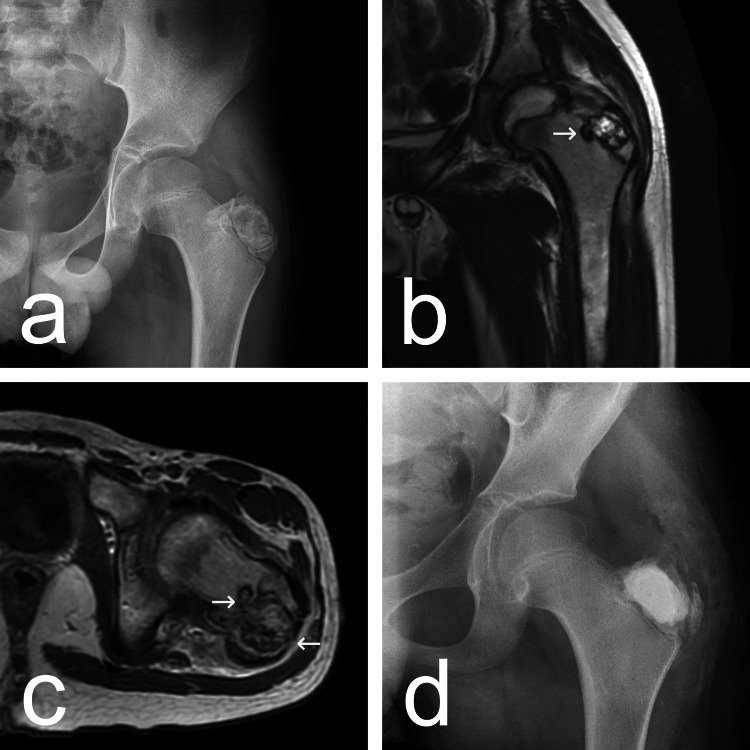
Imaging studies before and after surgery. 1a: Plain radiograph (anteroposterior) of the proximal femur at presentation. 1b, 1c: Frontal oblique and axial T1 weighted magnetic resonance tomography (MRT) images of the proximal femur at presentation. The growth plate and the lateral cortex are breached (arrows). 1d: Plain radiograph (anteroposterior) of the proximal femur after surgery.
